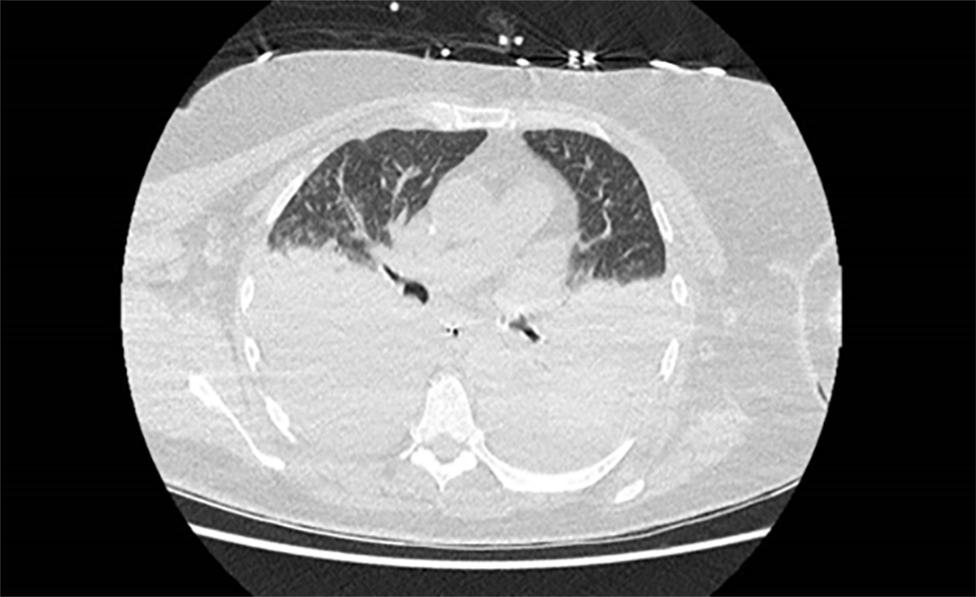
Axial computed tomography image from a case of autochthonous human *Babesia divergens* infection, England. Image shows bilateral pleural effusions.
